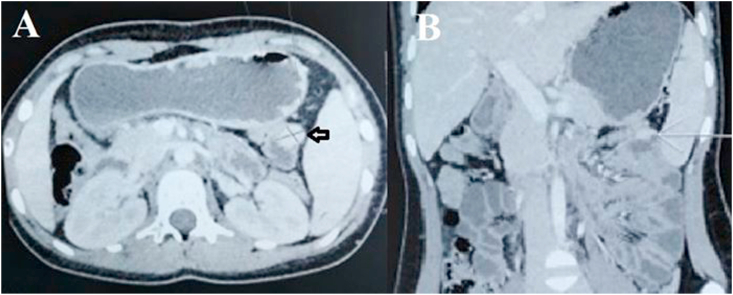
Contrast enhanced computed tomography showing the heterogeneously enhancing lesion in the jejunal wall (arrow) on axial (A) and coronal (B) sections.
